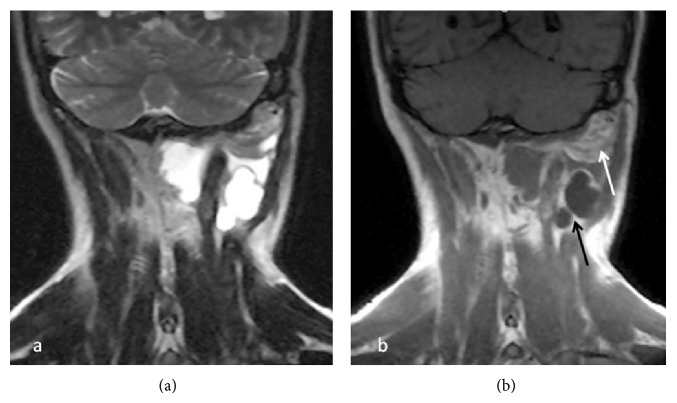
MRI findings of a 38-year-old male showed a multilobular cystic mass that was 8 × 5 × 4 cm and extended from the mastoid bone's posterior border along the sternocleidomastoid muscle to the larynx and was hyperintense on T2-weighted images (a) and hypointense on postcontrast T1-weighted images (b).
